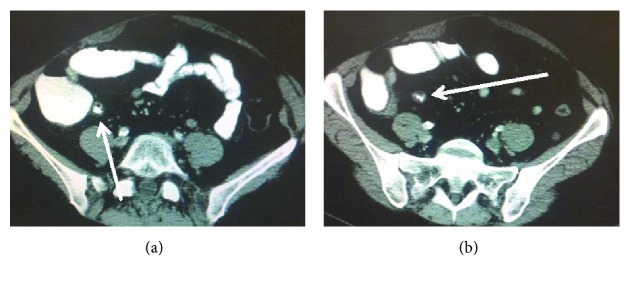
(a, b) CT scan: signs of appendicitis (stranding, diameter 9 mm), suspicious soft tissue masses.
